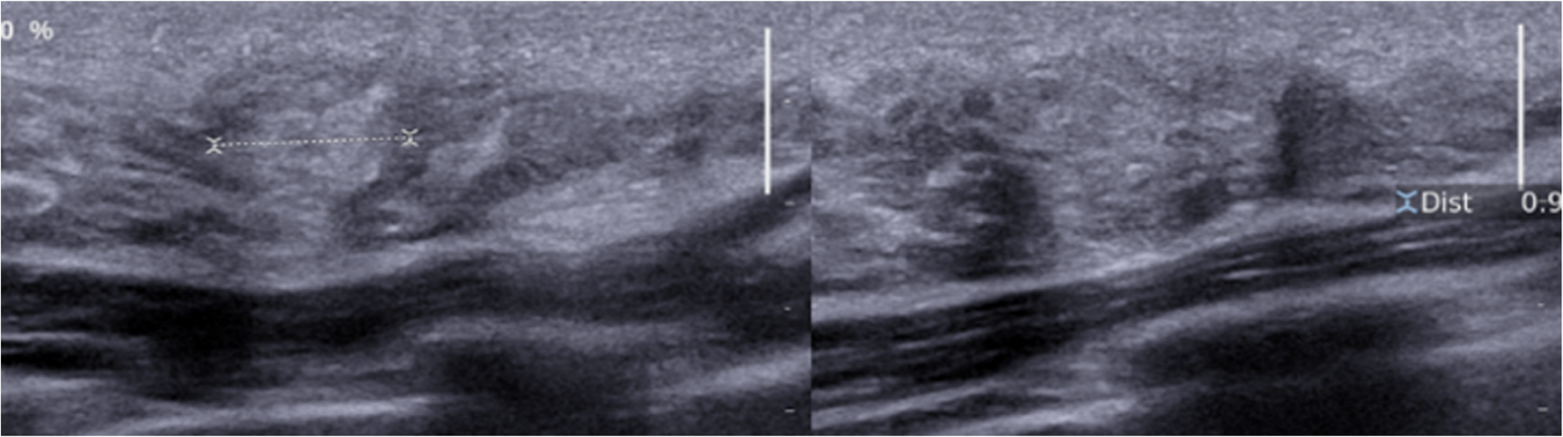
Complex sclerosing lesions. Ultrasound in a young woman with a palpable mass, revealing a hyperechoic zone with irregular margins and acoustic shadow. Microbiopsy demonstrating benign complex sclerosing lesions comprising duct structures and hyperplastic epithelium with no suspicious atypia, together with several pseudopapillary structures, and locally inflammatory periductal fibrosis
